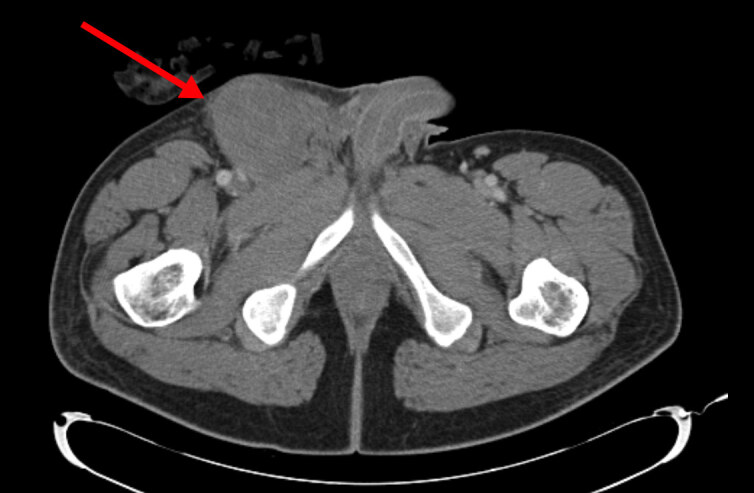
Computed tomography demonstrating a large heterogeneous, mass-like process within the right groin, which may be related to an unusually large hematoma.
